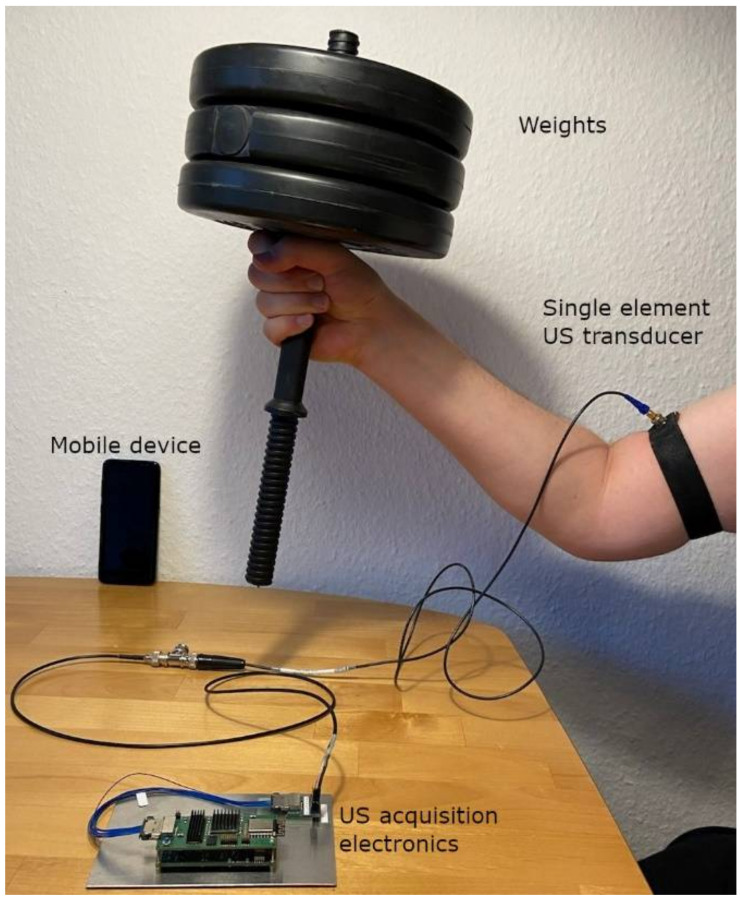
Experimental setup showing a participant lifting a weight, while a single element ultrasound transducer was attached to the body surface via a stretch armband. The signals were acquired with our custom-made acquisition hardware, which transfers them wirelessly to a mobile device.
